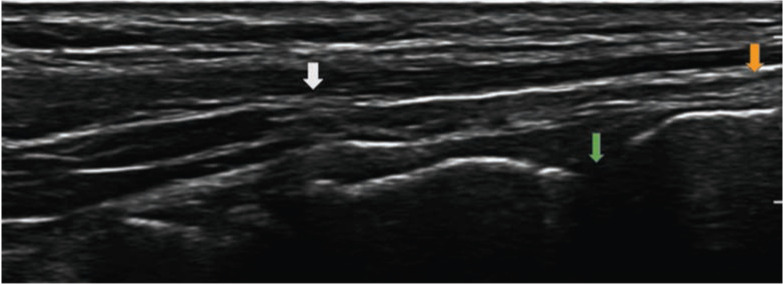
Ultrasound findings of the hallux. Tear in the extensor hallucis longus (EHL) tendon with retraction proximal to the interphalangeal joint up to the mid proximal phalanx (white arrow). Fluid gap at the dorsal lip of the left 1st distal phalangeal base where the EHL is expected to insert (orange arrow).
